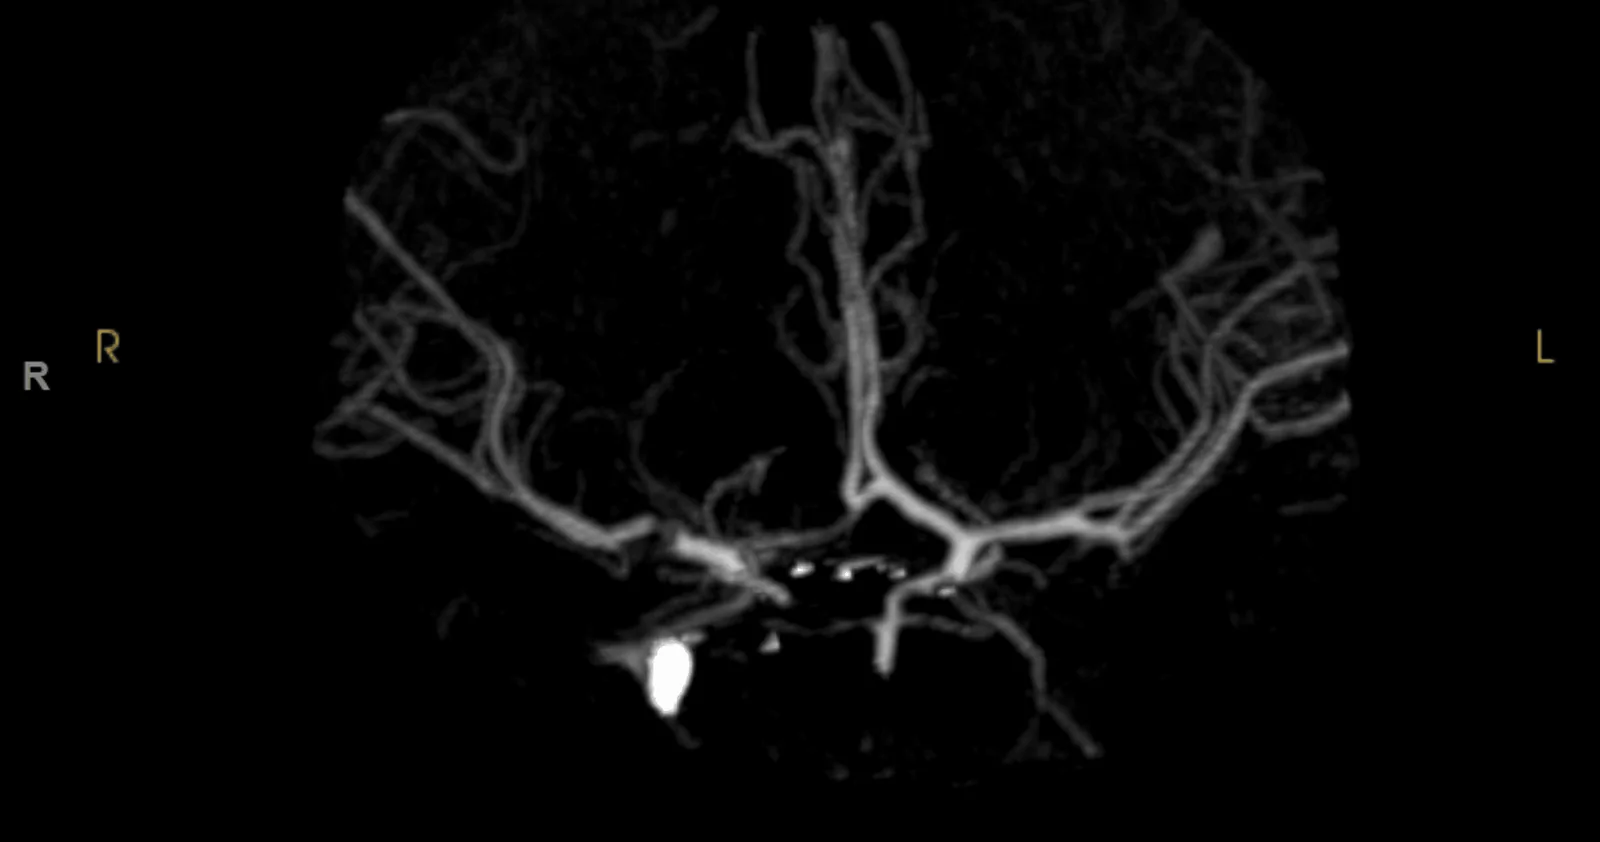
Admission head CT angiography CT angiography demonstrating a right MCA M1 segment occlusion CT: computed tomography, MCA: middle cerebral artery
